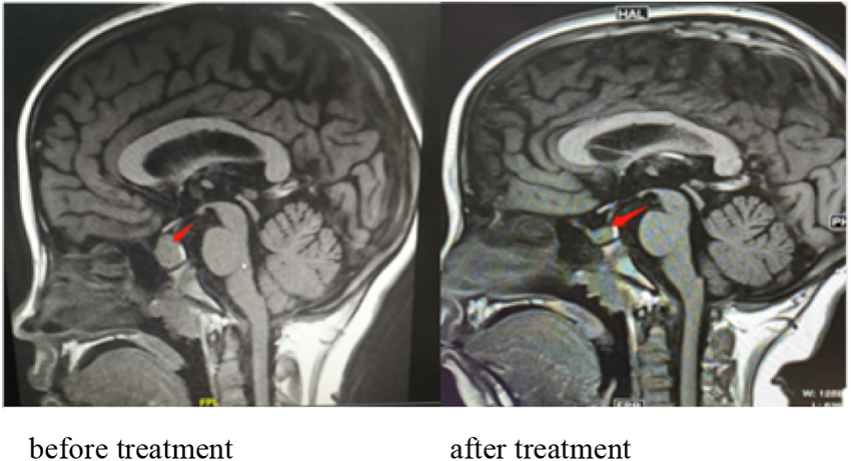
Pituitary MRI showing a mass in the sellar region with a uniform signal and a maximum height of about 15.8 mm. The pituitary stalk is still in the middle, without thickening. MRI showing that the pituitary decreased to 6.2 mm and returned to normal after treatment.
